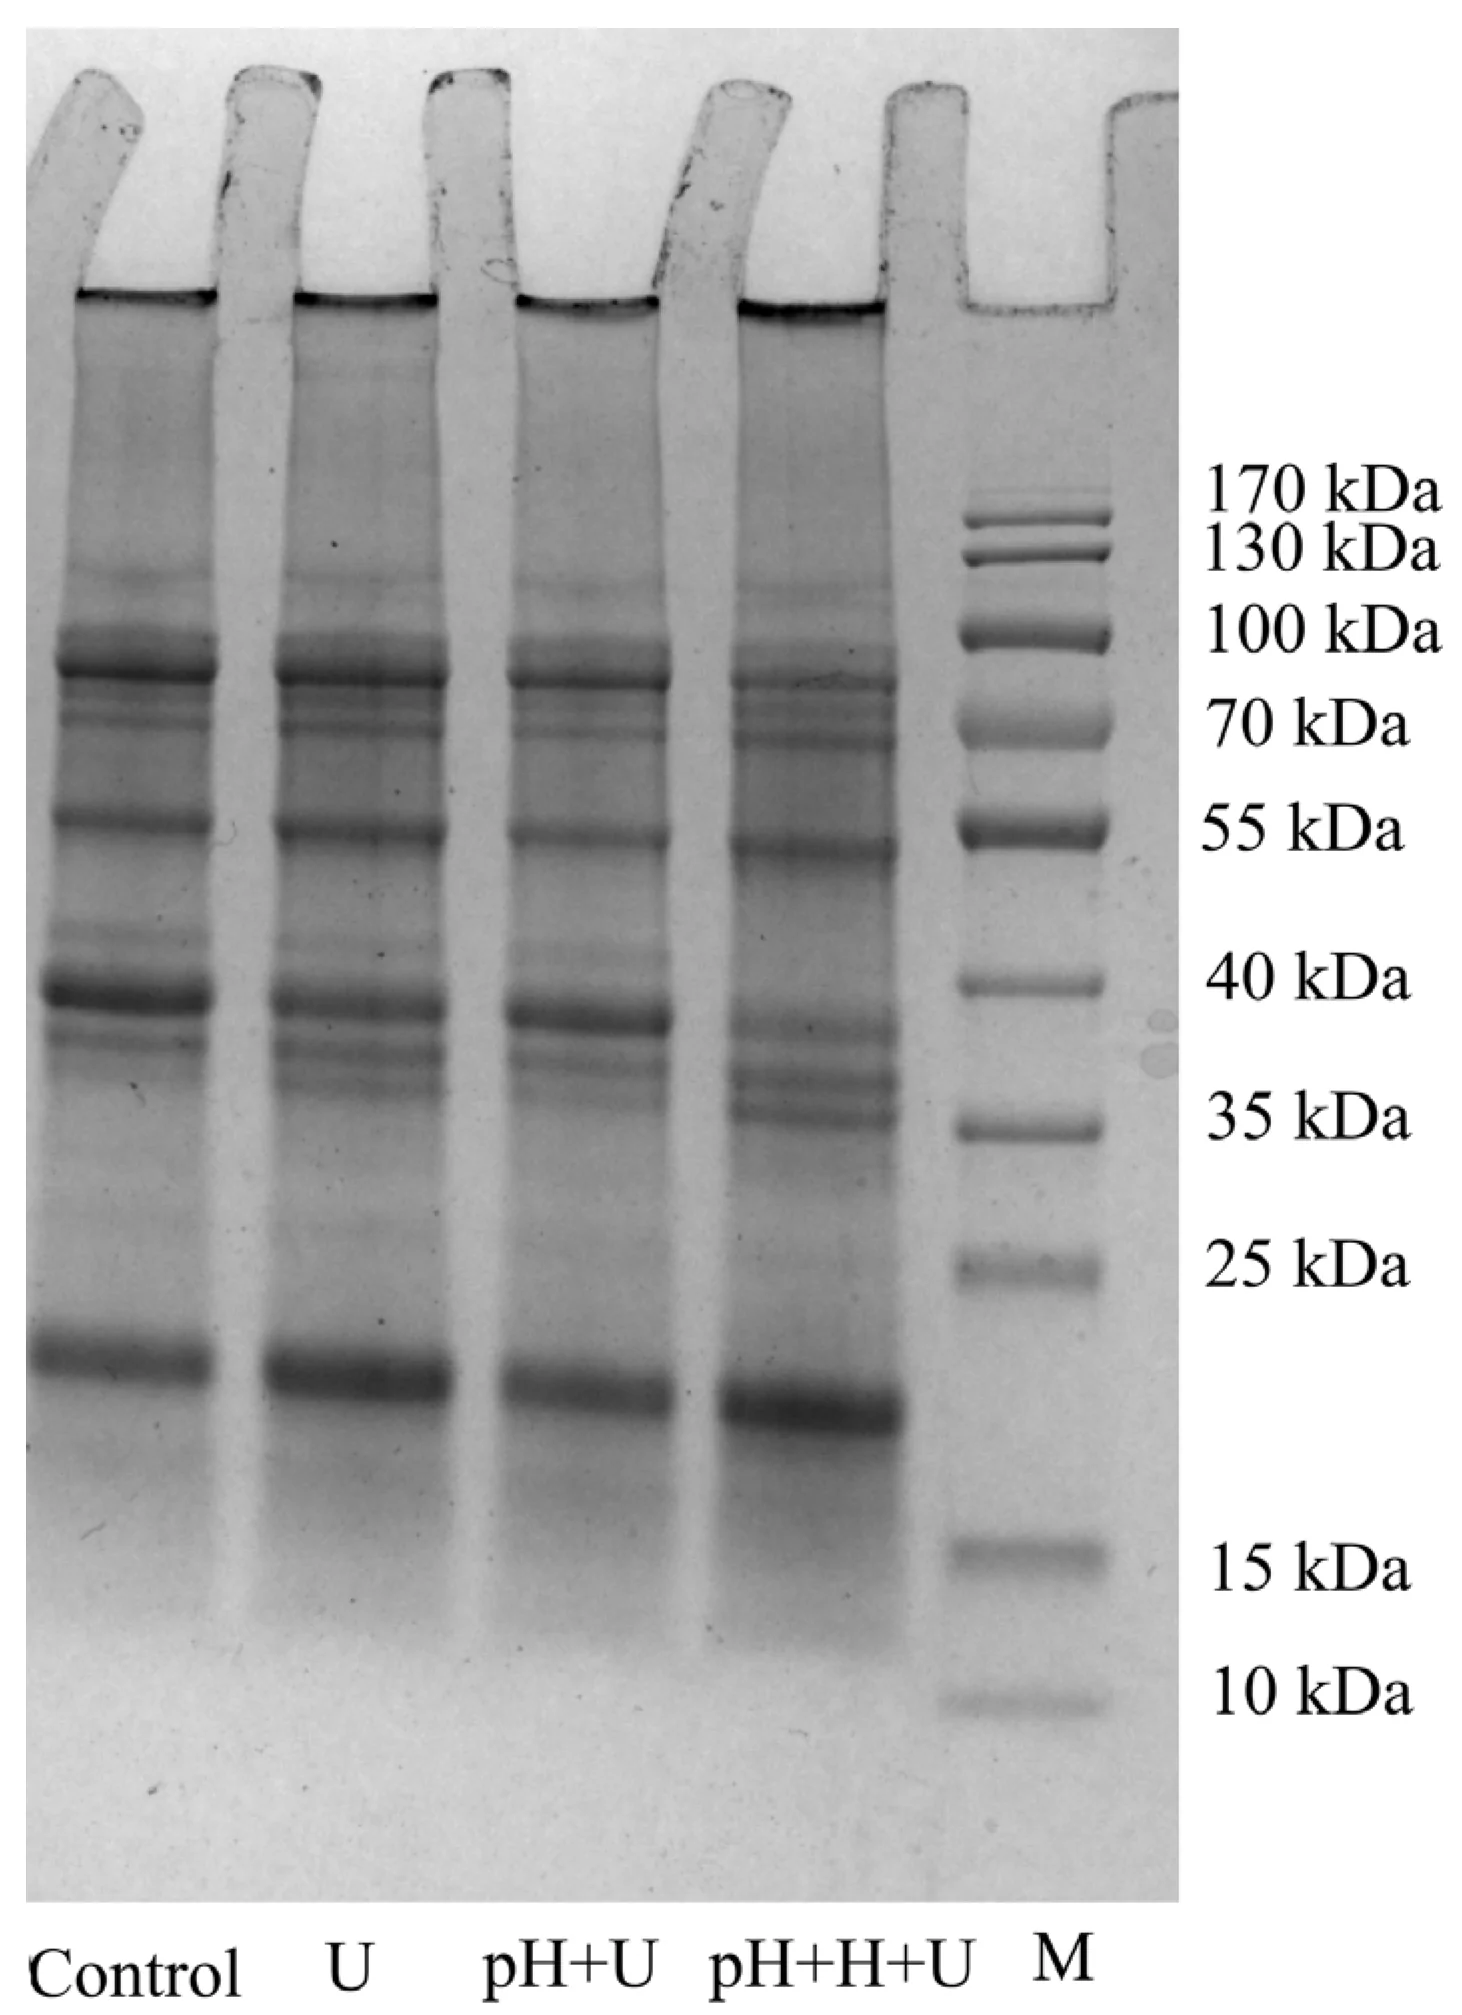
Electrophoretic profiles of perilla seed protein subjected to high-pressure homogenization, pH-shifting, and ultrasound treatments. Treatment labels are the same as in Figure 1.
